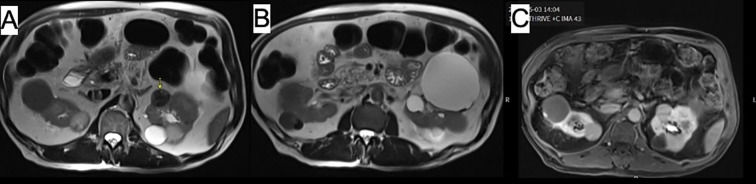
Abdominal MRI images showing (A, B) mass within anterior upper pole of left kidney with thick irregular internal septation (arrow). Image (C) demonstrates additional cysts in both kidneys.
